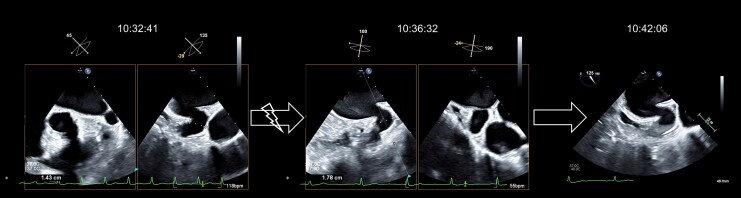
Transoesophageal view of a pre-thrombotic formation in the LAA after electrical cardioversion.
